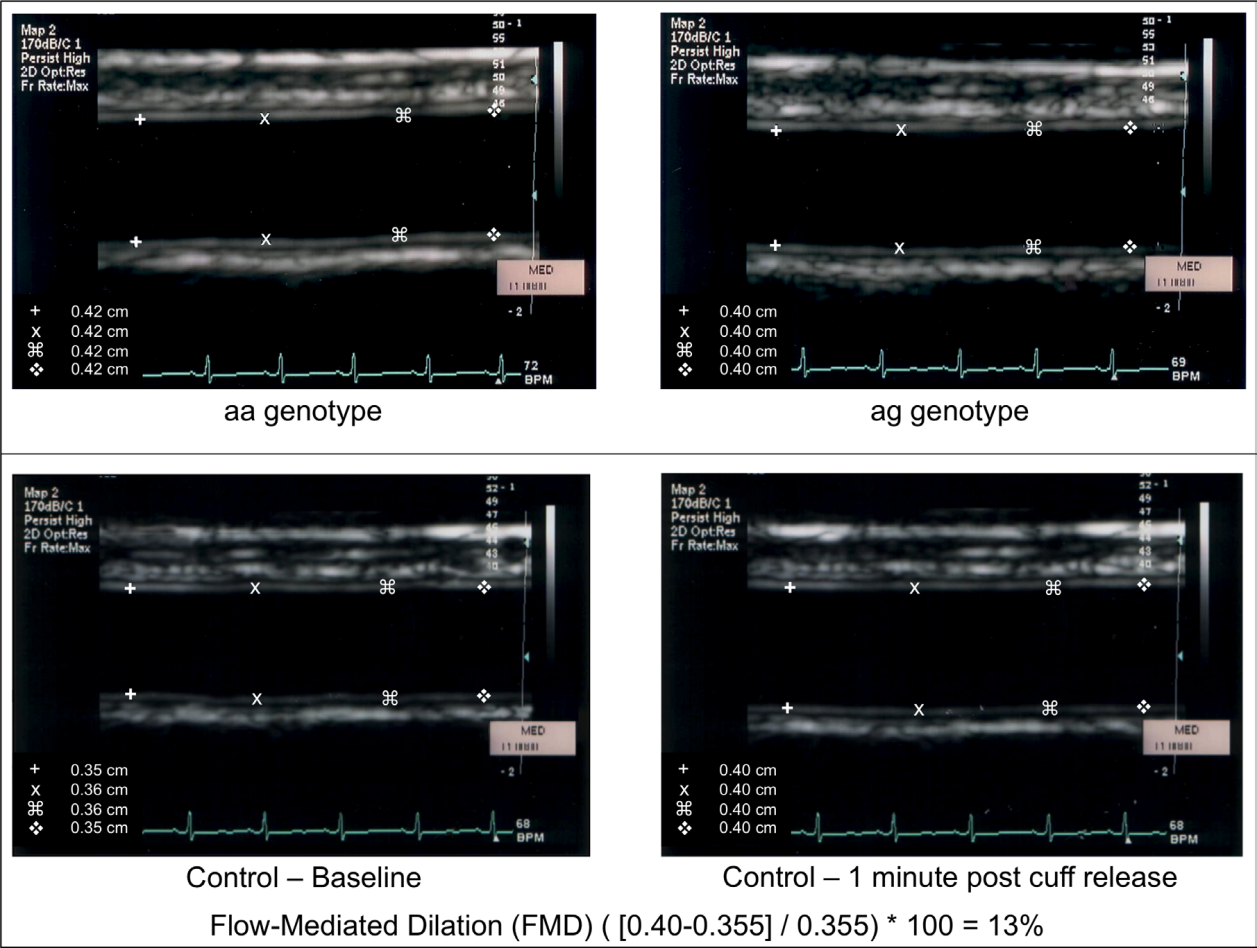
Representative images of the baseline brachial ultrasound of a participant with the aa genotype (top left picture), of a participant with the ag genotype (top right picture), and of the baseline and post-hyperemic ultrasound of a healthy control (bottom pictures). The calculation of flow-mediated dilation (FMD) using the values collected in the healthy control is also reported.
